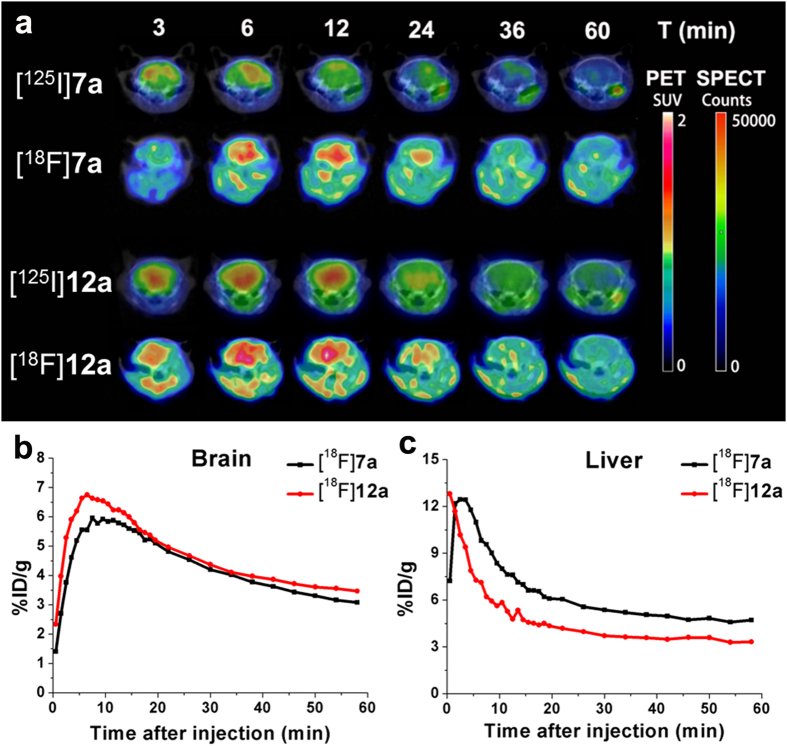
Dynamic microPET/CT and microSPECT/CT imaging. (a) Transversal brain SPECT images of [^125^I]7a, [^125^I]12a and PET images of [^18^F]7a, [^18^F]12a superimposed onto CT templates. SPECT image color intensities are expressed as counts, while PET images represent SUV. Upper row indicates time points of image acquisition. (b,c) TACs of [^18^F]7a and [^18^F]12a in brain and liver for the entire 60 min PET scan.
